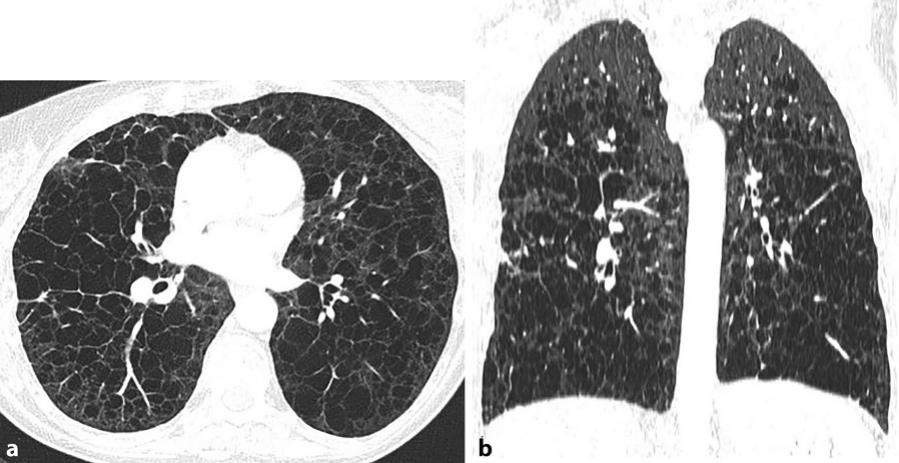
CT einer 58-jährigen Patientin mit bekannter Lymphangioleiomyomatose (LAM) zur Abklärung einer möglichen Lungentransplantation. a Axiales Lungenfenster, b koronale Rekonstruktion im Lungenfenster. Bild einer ausgedehnten zystischen Lungenerkrankung mit nur noch wenig residuell verbleibendem Lungenparenchym, insbesondere in den Mittel- und Unterlappen führend. Die Lungenzysten zeigen im Vergleich zur Langerhans-Zell-Histiozytose (LZH; s. Abb. 4 in „Teil 1“) eine dünnwandige, rundlich-homogene Kontur, während die Zystenkonfiguration bei der LZH unregelmäßig und bizarr erscheint. Das Bild könnte leicht mit einem Lungenemphysem verwechselt werden, wobei eine fehlende – für das Emphysem jedoch typische – apikale Prädominanz hier differenzialdiagnostisch hilfreich sein kann. Bei der Patientin sind keine Angiomyolipome bekannt
